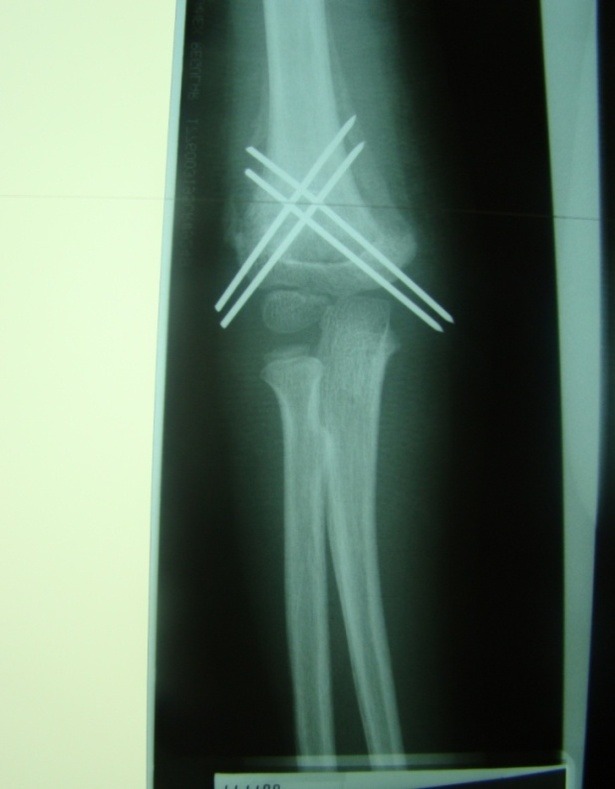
X-ray image of an hypertrophic callus formed after an old, neglected fracture was treated using the open technique.
